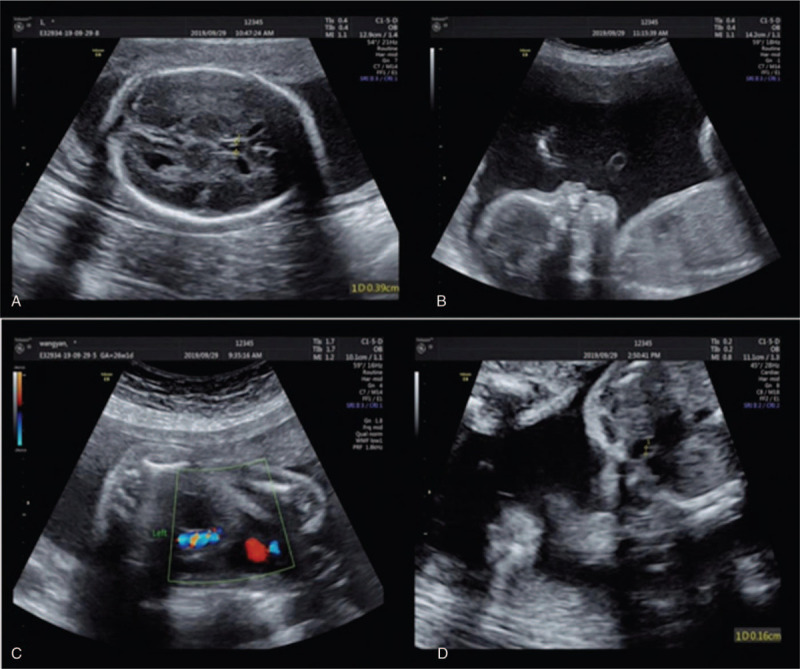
Prenatal ultrasound at 20 wk of gestation shows the fetus with an acoustic image of a transparent septum (A), absence or hypoplasia of the nasal bone (B), a single umbilical artery (C), and right pulmonary artery stenosis (D).
